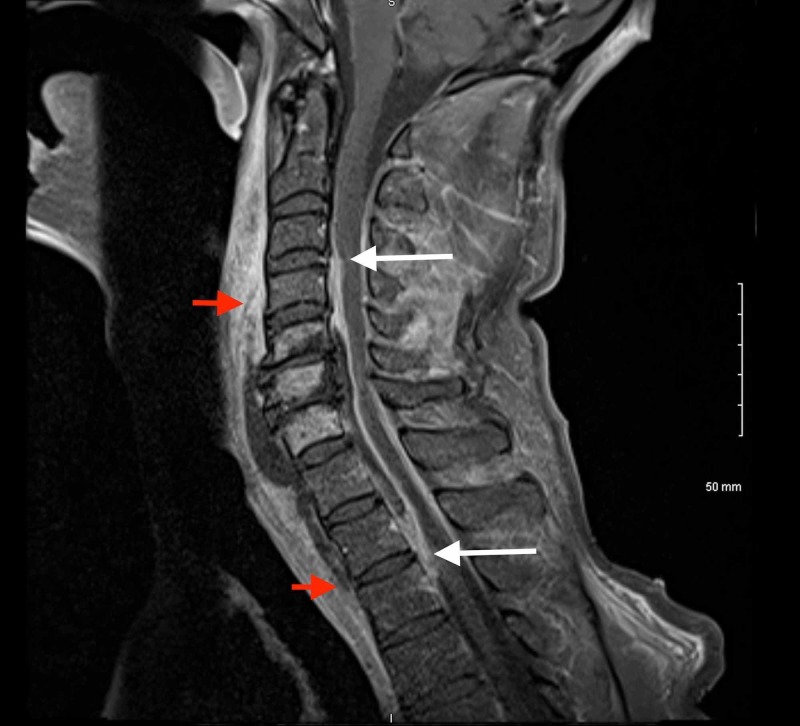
Sagittal T1 post-contrast image of the cervicothoracic spine showing a fluid collection in the anterior epidural space from C3 to T2 (white arrows), and prevertebral fluid collection from C4 to T2 (red arrows). MRI = magnetic resonance imaging
